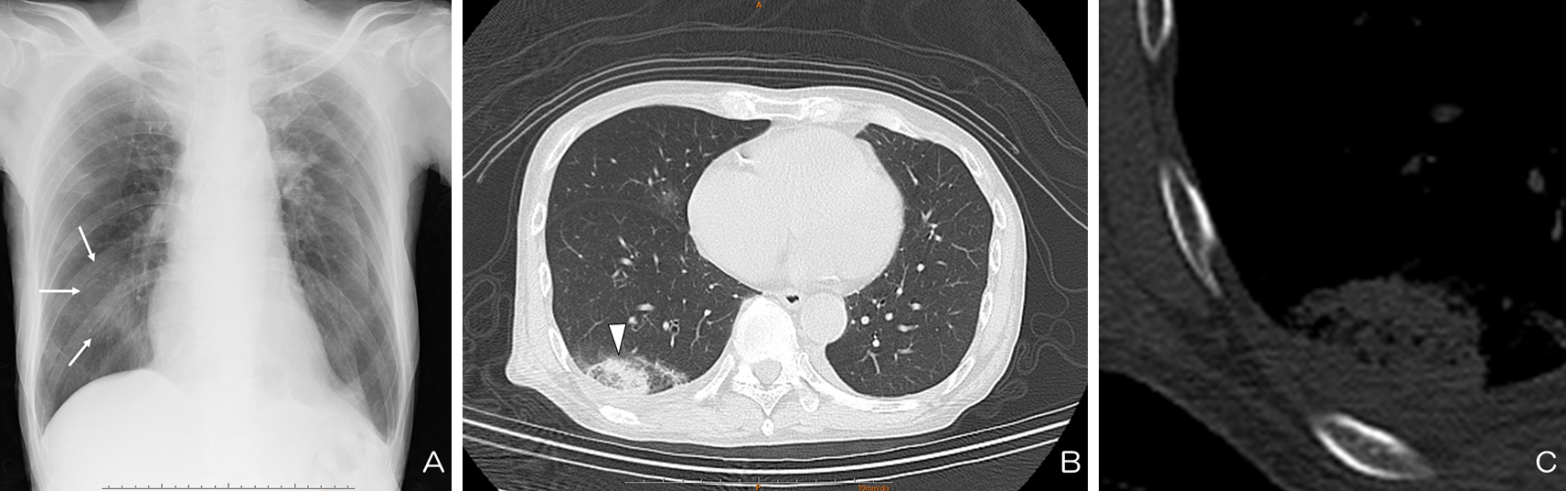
(A) Slight consolidation in chest X-ray (arrow) (B) Isolated patchy consolidation adjacent with the pleura in computed tomography (arrowhead) (C) A wedge-shaped consolidation without the expected apex seen well in the mediastinal. A possible reason of sparing the apex is collateral supply from the bronchial arterial circulation.
